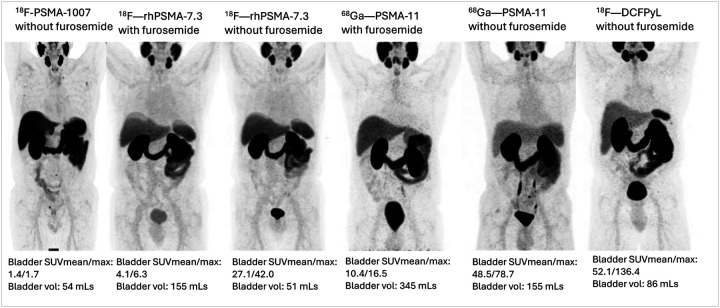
MIP images with similar windowing level showing relative bladder activity of different PSMA radioligands on PET imaging acquired with or without furosemide administration.
